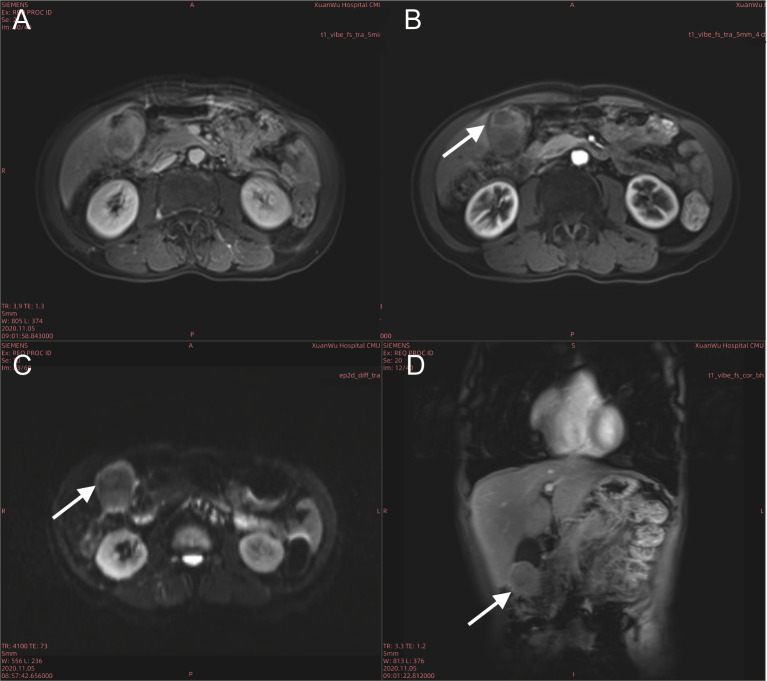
Representative MR findings of the hepatoid adenocarcinoma. (A) Axial MR image showing a round abnormal signal at the gallbladder fundus. (B, D) Axial and coronal contrast-enhanced MRI in the arterial phase showing early enhancement of the mass (white arrows), mimicking the hemodynamic pattern of hepatocellular carcinoma. (C) Diffusion-weighted image showing restricted diffusion, reflecting the aggressive nature of the lesion.
